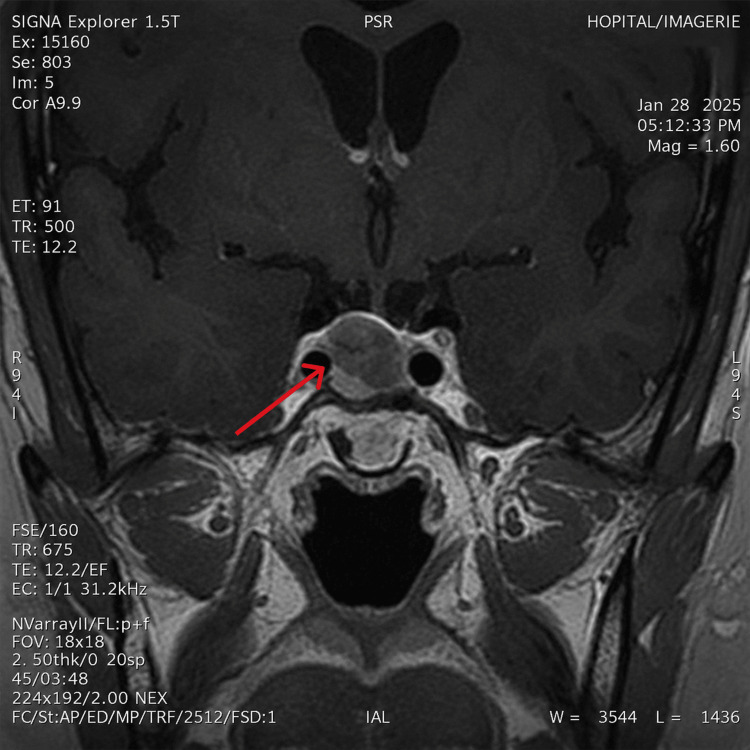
Magnetic resonance imaging of the pituitary region, coronal section, necrotic-hemorrhagic sella turcica measuring 14 x 15 x 12 mm.
